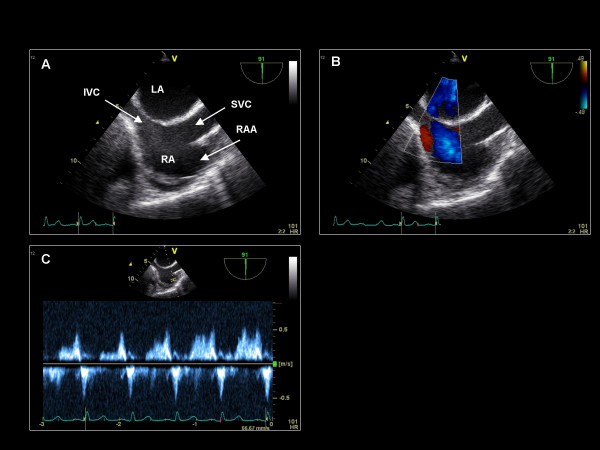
Imaging plane 90°, (A) The bicaval view demonstrating the SVC, inferior vena cava (IVC), and RAA, and interatrial septum. (B) Color Doppler applied to the interatrial septum. (C) Pulse wave Doppler of the RAA demonstrating normal emptying velocities in a patient in sinus rhythm.
